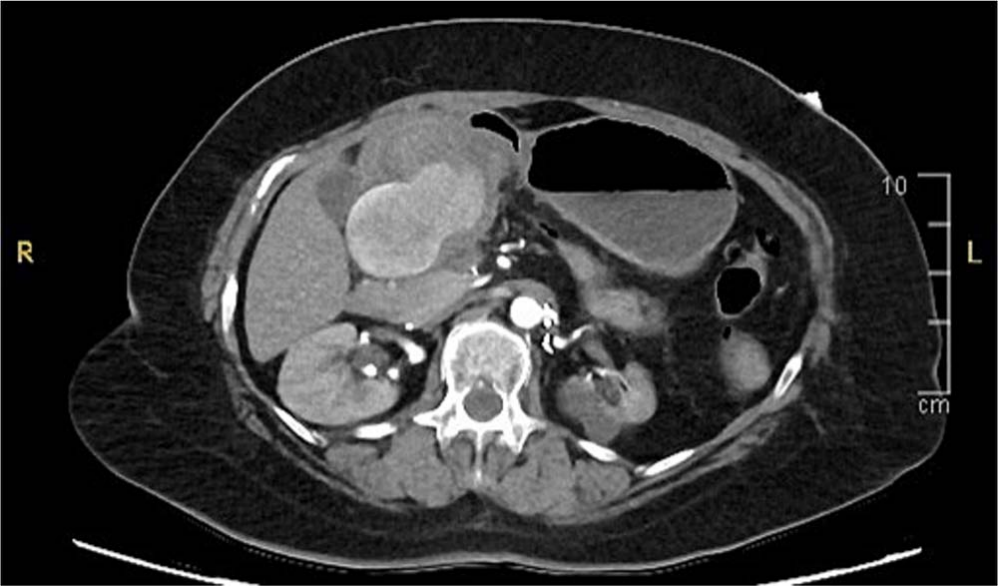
Contrast-enhanced CT angiography of the abdomen. Axial view showing a large 6.2 × 4.2 × 7.0 cm pseudoaneurysm arising from the gastroduodenal artery.
